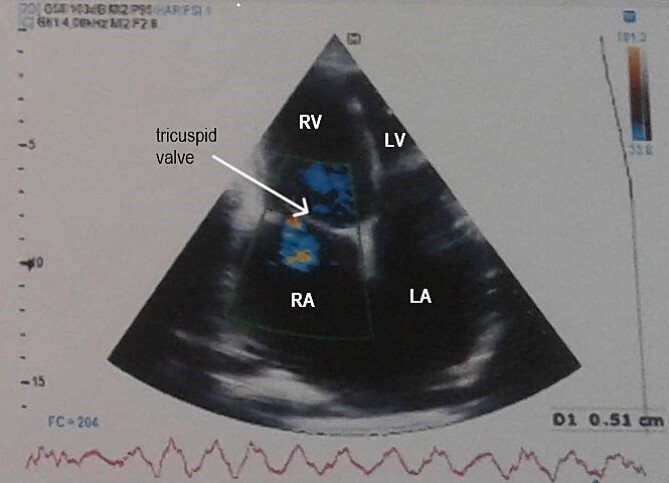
Transthoracic echocardiography showed pericardial thickening, moderate tricuspid regurgitation and biatrial dilation. RA: right atrium, RV: right ventricle, LA: left atrium, LV: left ventricle.
